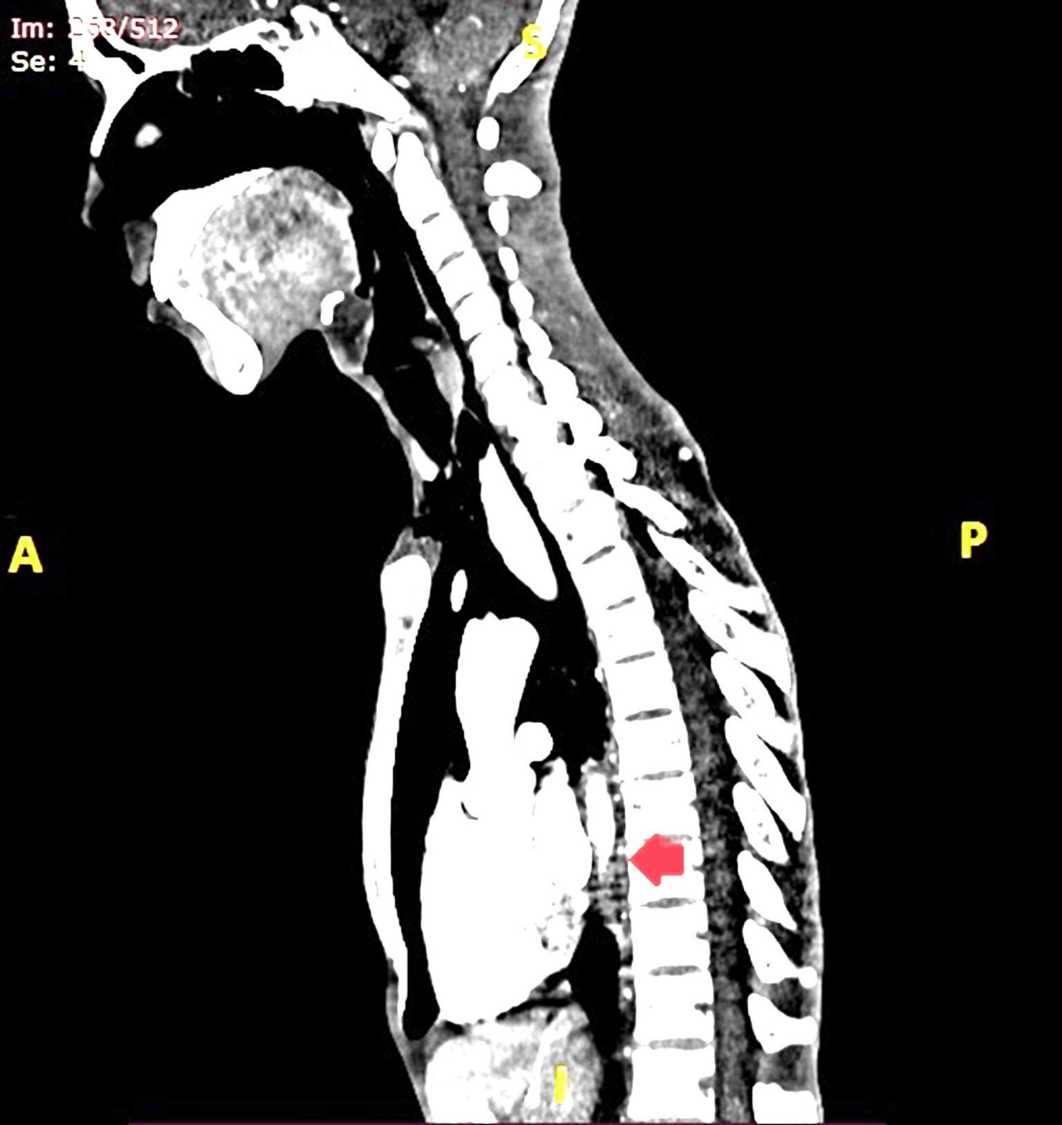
Sagittal section CECT scan of the thorax showing lesion involving the middle and lower third of the esophagus with contiguous involvement of the GEJ, extending from D7 to D11 vertebral segments and completely occluding the lumen (Red Arrow). CECT: contrast-enhanced computed tomography; GEJ: gastroesophageal junction; D7 to D11: dorsal vertebrae 7th to 11th
